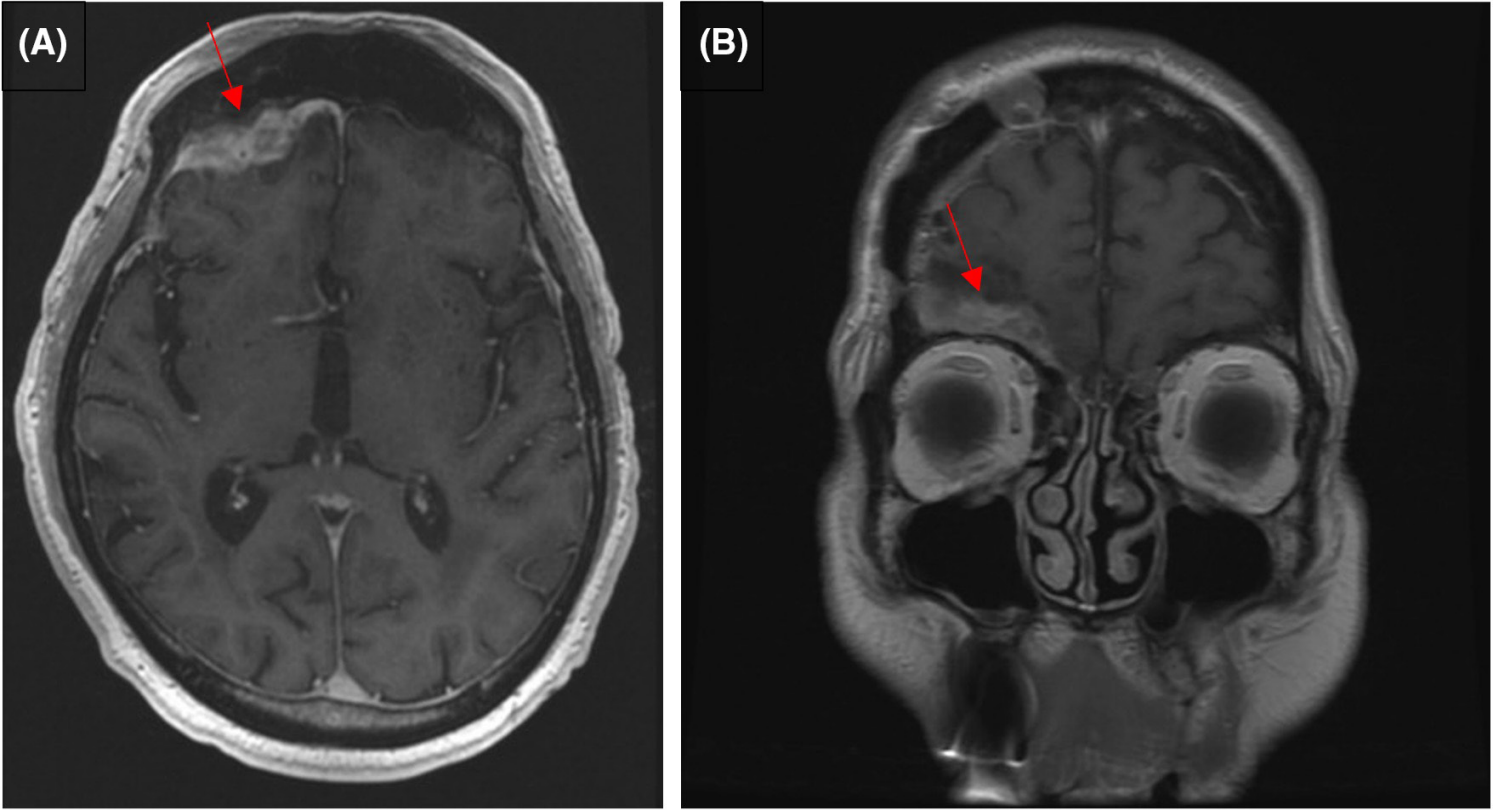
March 19, 2017 (1 month post–chemotherapy). MRI head post gadolinium 3D T1 axial and sagittal views showing thick lobulated enhancement of the dural margin increasing in size to 4.5  × 1.4 cm, felt to represent residual tumor mixed with reactive dural enhancement.
